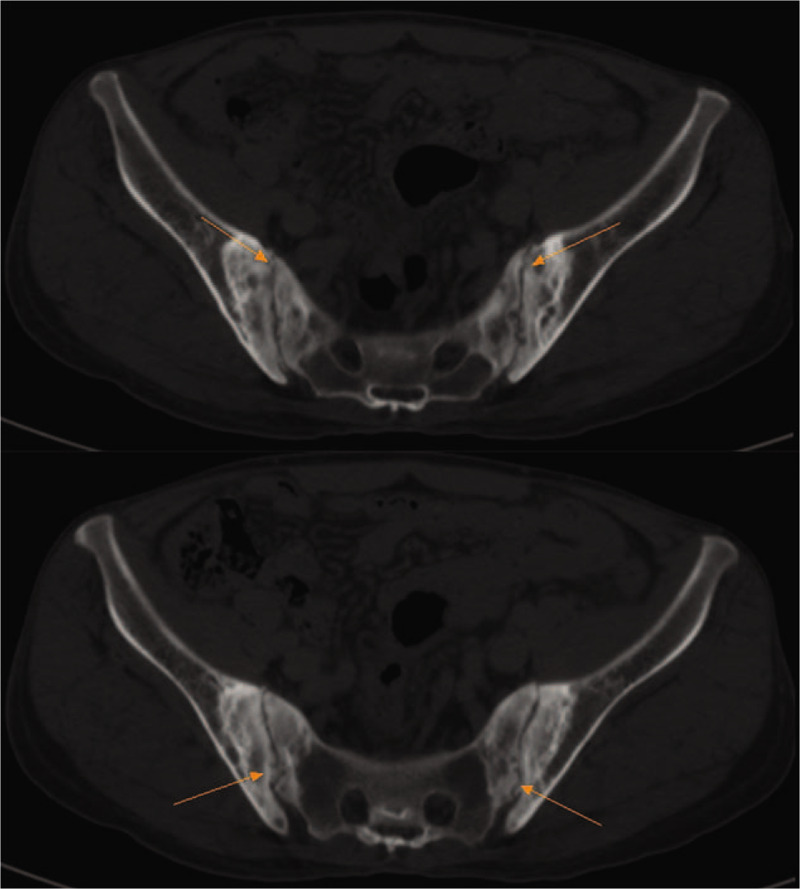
Sacroiliac joint CT revealed narrowing of bilateral sacroiliac joints space and serrated margin, multiple cystic destruction, hyperosteogeny and sclerosis of articular surface.
